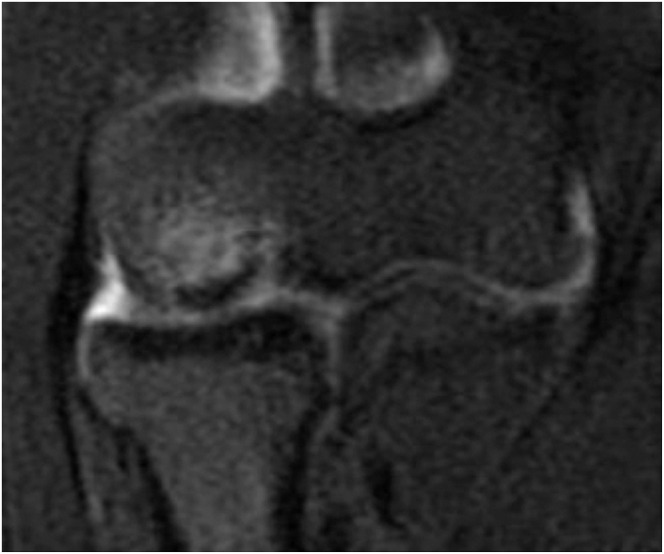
Coronal view of a T2 MRI sequence of left elbow showing a large (>14 mm) unstable capitellar OCD lesion. (MRI, magnetic resonance imaging; OCD, osteochondritis dissecans.)
